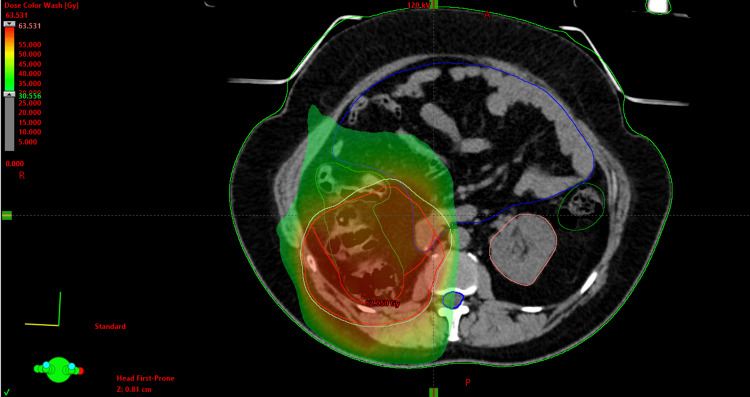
Representative axial CT slice of adjuvant radiotherapy plan, which the patient received after her first surgery. The tumor bed received up to 60 Gy. The maximal point dose received by the large bowel, duodenum, and spinal canal were 62.36, 62.38, and 50.24 Gy, respectively
